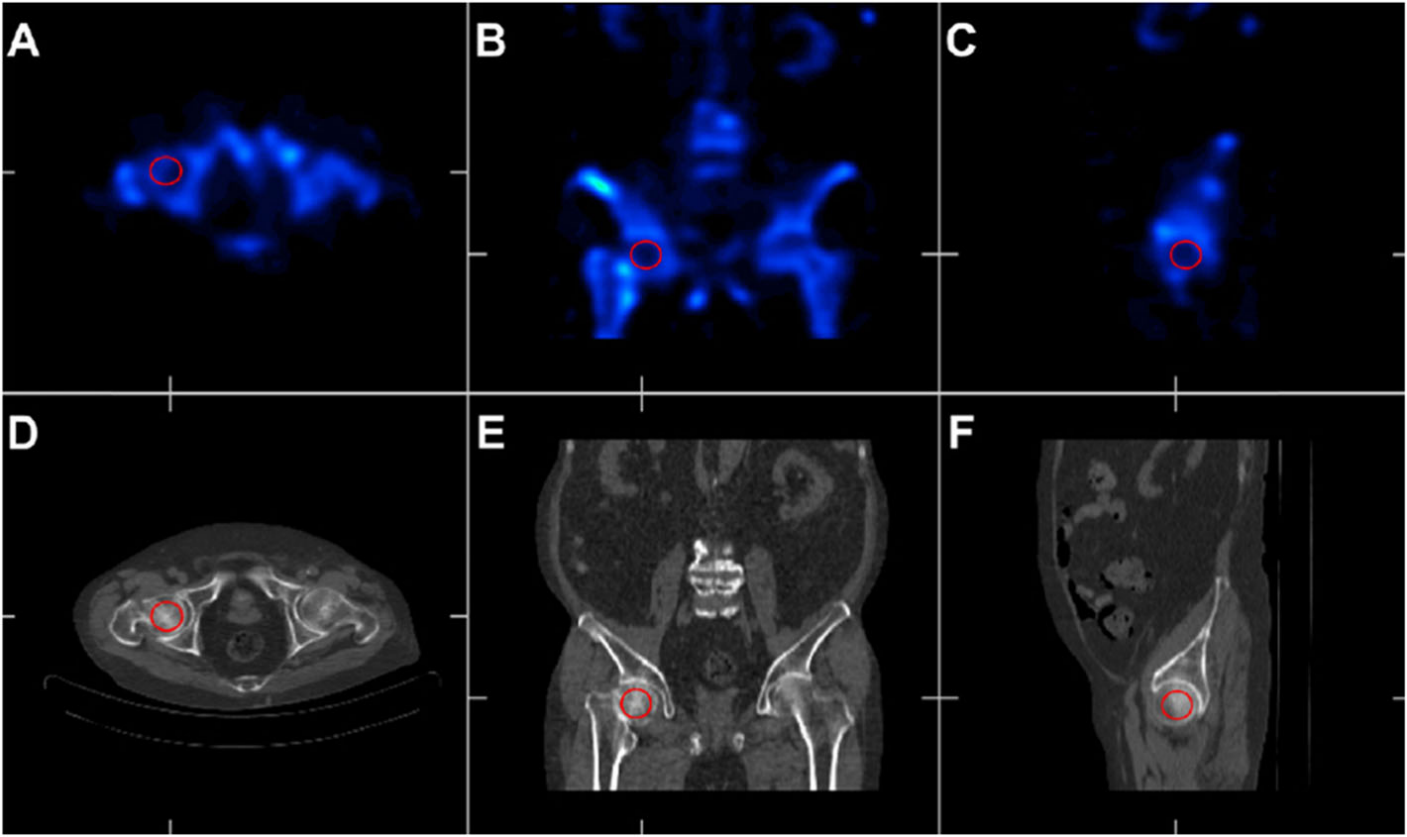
Quantitative analysis of the head-to-head uptake ratio with ROI in a 3-cm circle, excluding the cortical bone of femur head. a,d ROI measured in axial view of SPECT/CT. b,e ROI in coronal view. c,f ROI in sagittal view. Three independently measured values of pixels are summated to an average value of ROI
